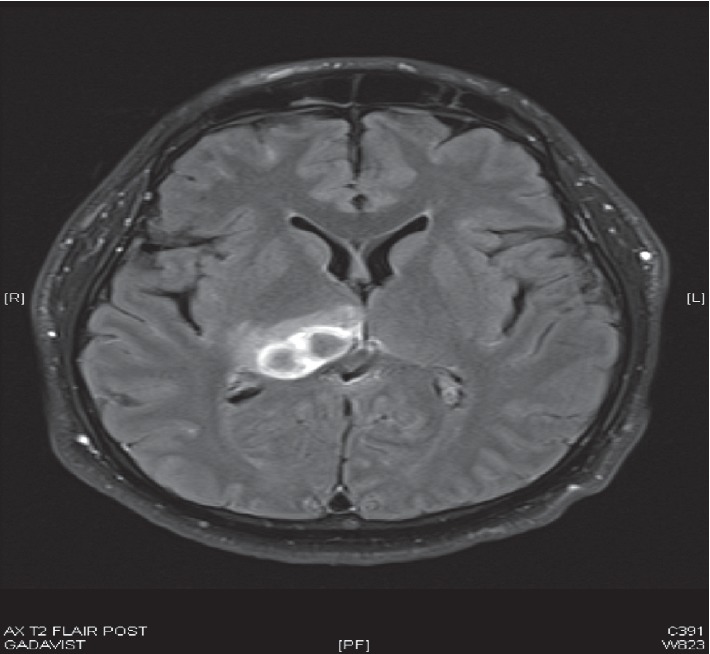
T2 flair MRI brain with contrast showing a 3 cm multiloculated cystic lesion (abscess) over the posterior thalamus with surrounding edema.
